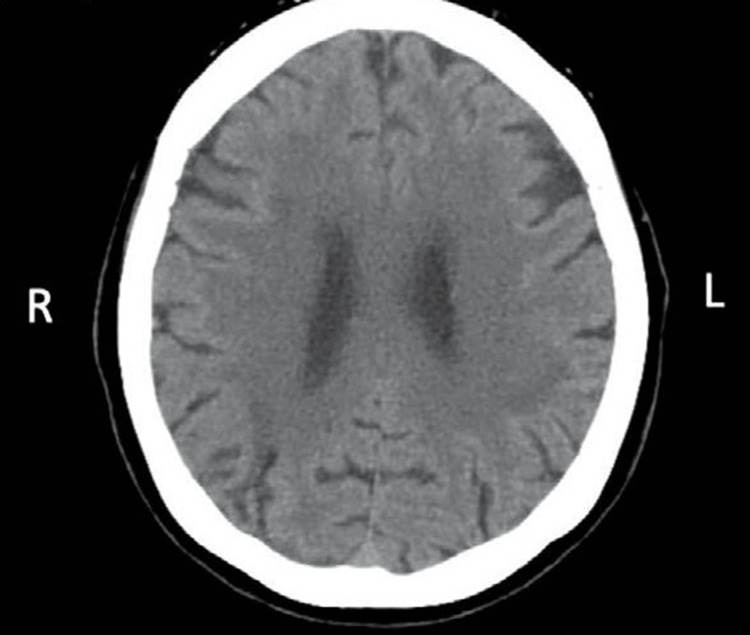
Computed tomography image of the brain Computed tomography revealed a low intensity in the surrounding cortex of the brain.
